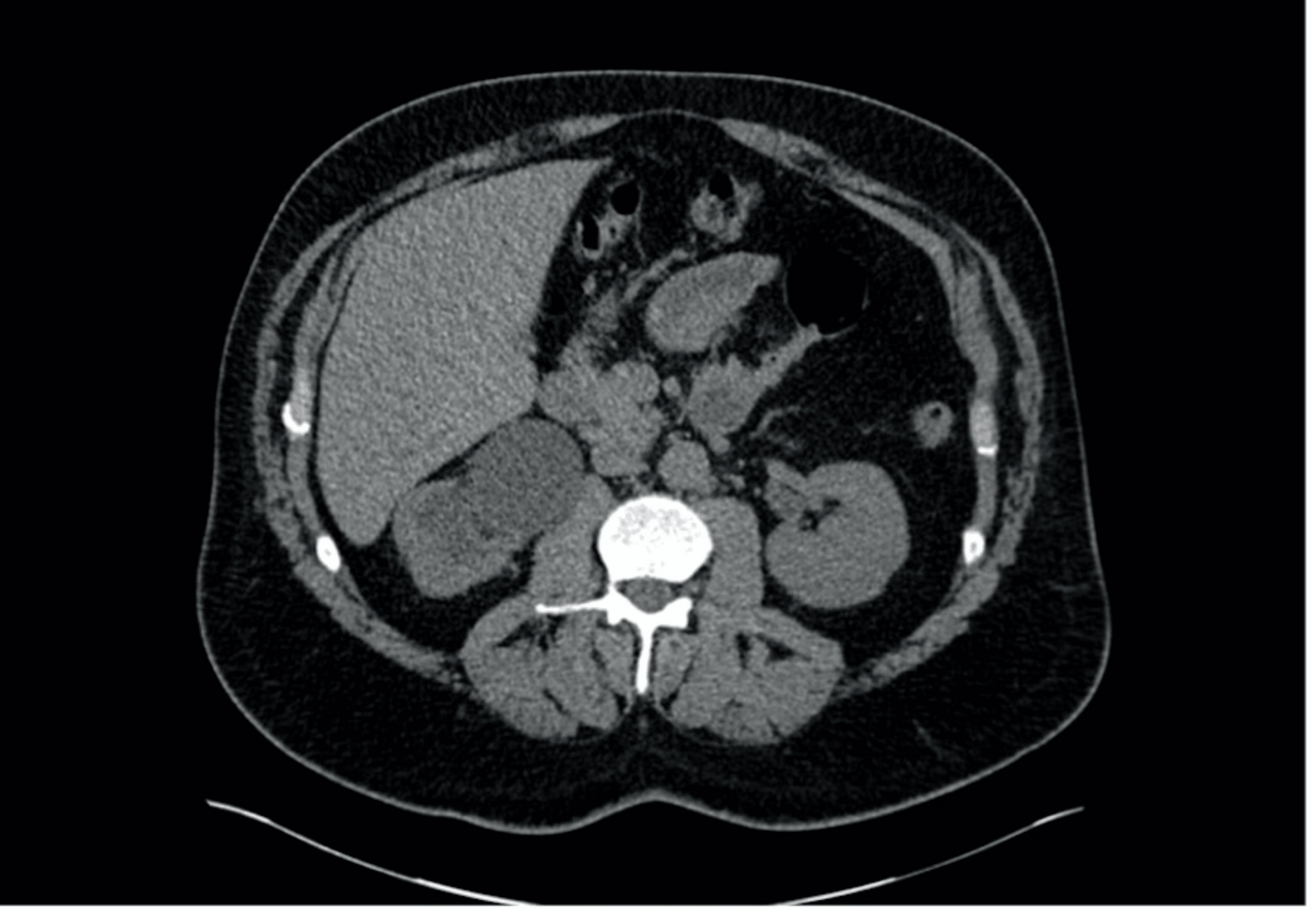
CT kidney ureter bladder (KUB) demonstrating severe chronic right hydronephrosis and proximal hydroureter with long segment thickening and stricture at the mid-distal ureter. Adjacent subcentimeter lymph nodes and periureteral fat stranding with moderate cortical thinning.
